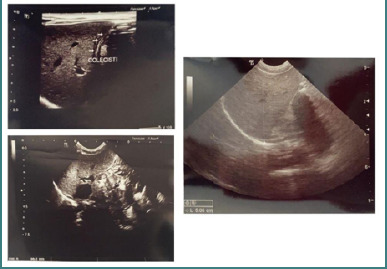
Abdominal ultrasound at 1 month of life. The liver demonstrates homogeneous echotexture, smooth surface contour, and normal size. The spleen appears enlarged with coarse echotexture, consistent with persistent clinical splenomegaly.
